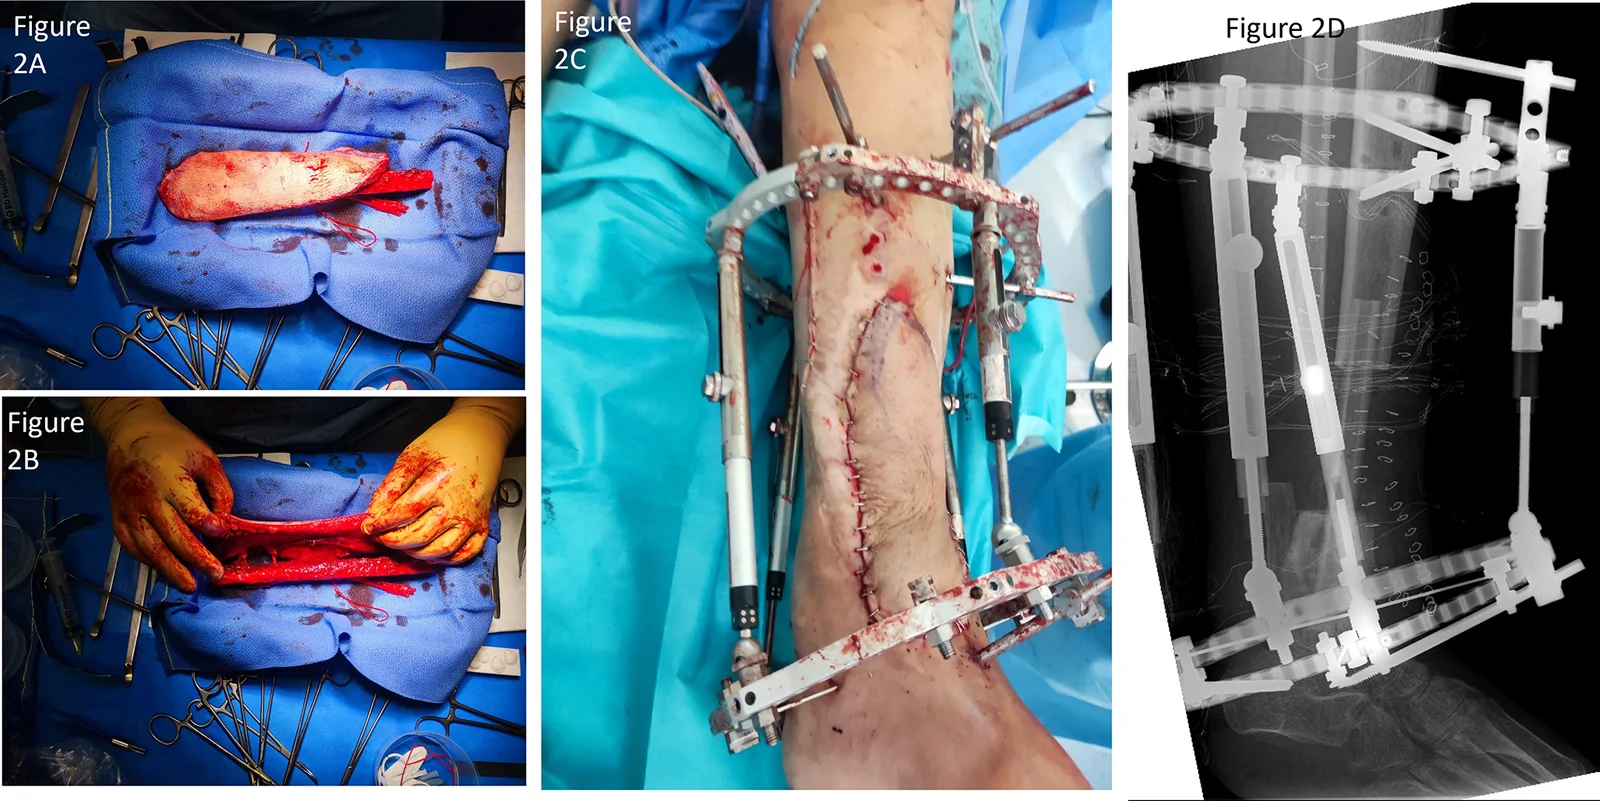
Representative case of vascularized fibular grafting (VFG) for infected non-union of the lower limb. (A) Harvest of a vascularized fibular osteocutaneous graft with skin paddle from the contralateral leg. (B) Isolated vascularized fibular graft after harvest. (C) Intraoperative view following implantation of the vascularized fibular graft at the recipient site. (D) Postoperative radiograph demonstrating graft positioning and fixation after VFG reconstruction.
